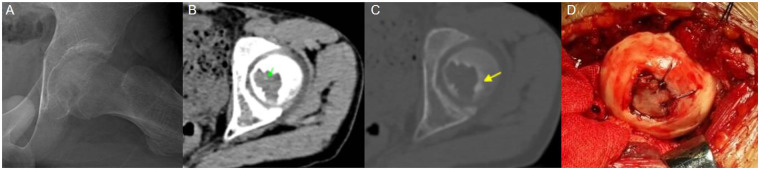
Case 3: (A) x-ray: a lucent low-density lesion is present in the medial aspect of the left femoral head. (B) CT (soft tissue window): Punctate calcifications (green arrow). (C) CT (bone window): a thin sclerotic margin surrounds the lesion.(yellow arrow). (D) The smooth cortical surface of the iliac bone was used to suture and repair the necrotic articular cartilage surface.
